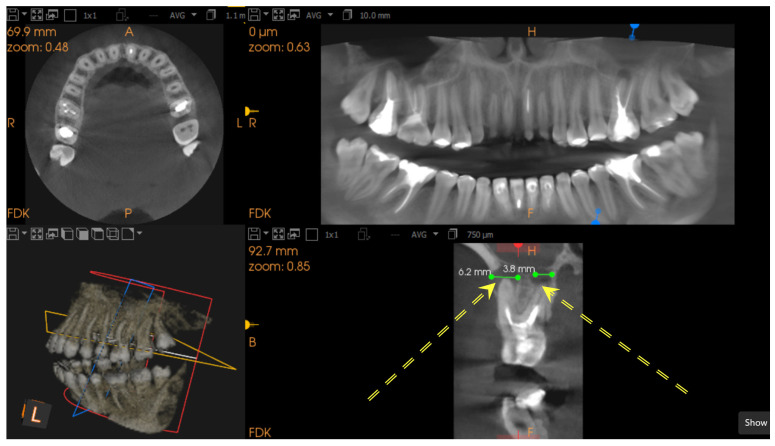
Multiplanar CBCT images of a maxillary molar with periapical radiolucency measuring 3.8 mm and 6.2 mm. The lesion (marked by double yellow arrows) is well-circumscribed and consistent with a CBCT-PAI score of 3–4. Views include axial, panoramic reconstruction, 3D rendering, and sagittal slice.
